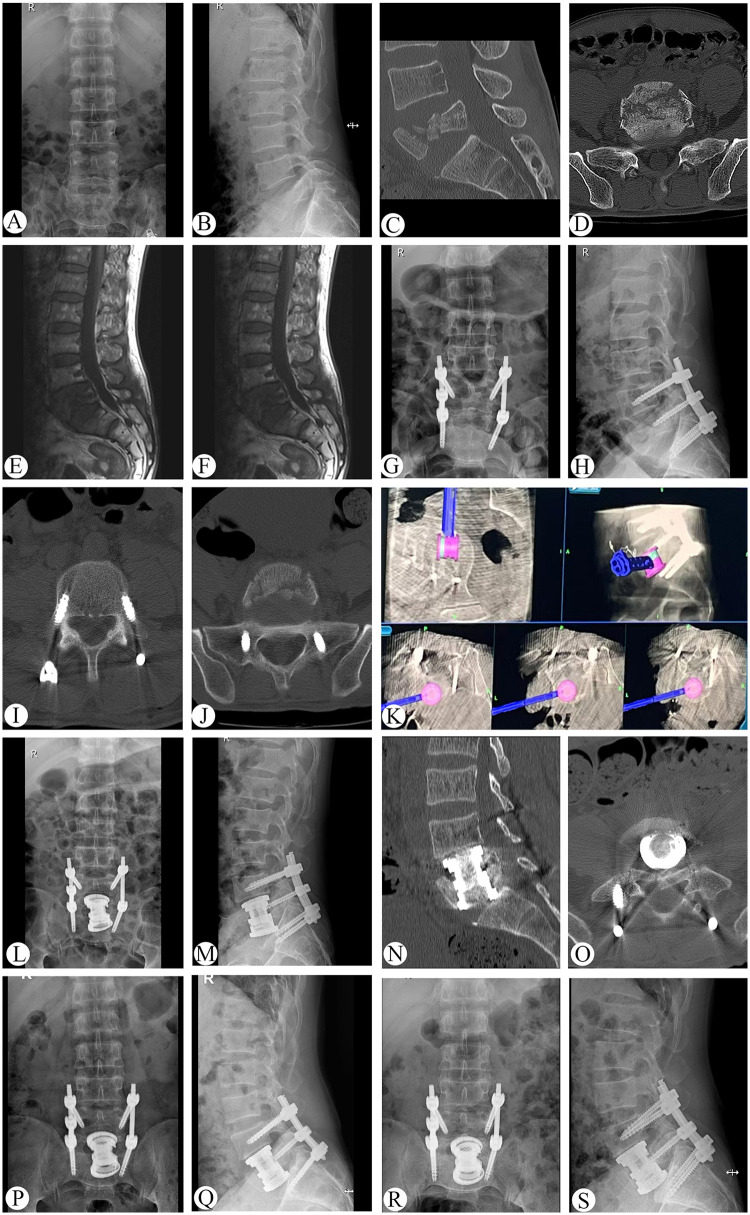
Preoperative, postoperative, and follow-up radiographs of a 21-year-old patient who had dated L5 vertebral fractures with kyphotic deformity managed by anterior vertebrectomy and reconstruction with O-arm navigation (case 15). (A–F) x-ray, CT, and MRI before operation. (G–J) x-ray and CT after posterior operation. (K) Real-time three-dimensional imaging of O-arm navigation. (L–O) x-ray and CT at 3 days after operation. (P,Q) x-ray at 1 years follow-up. (R,S) x-ray and CT at 2.7 years follow-up.
